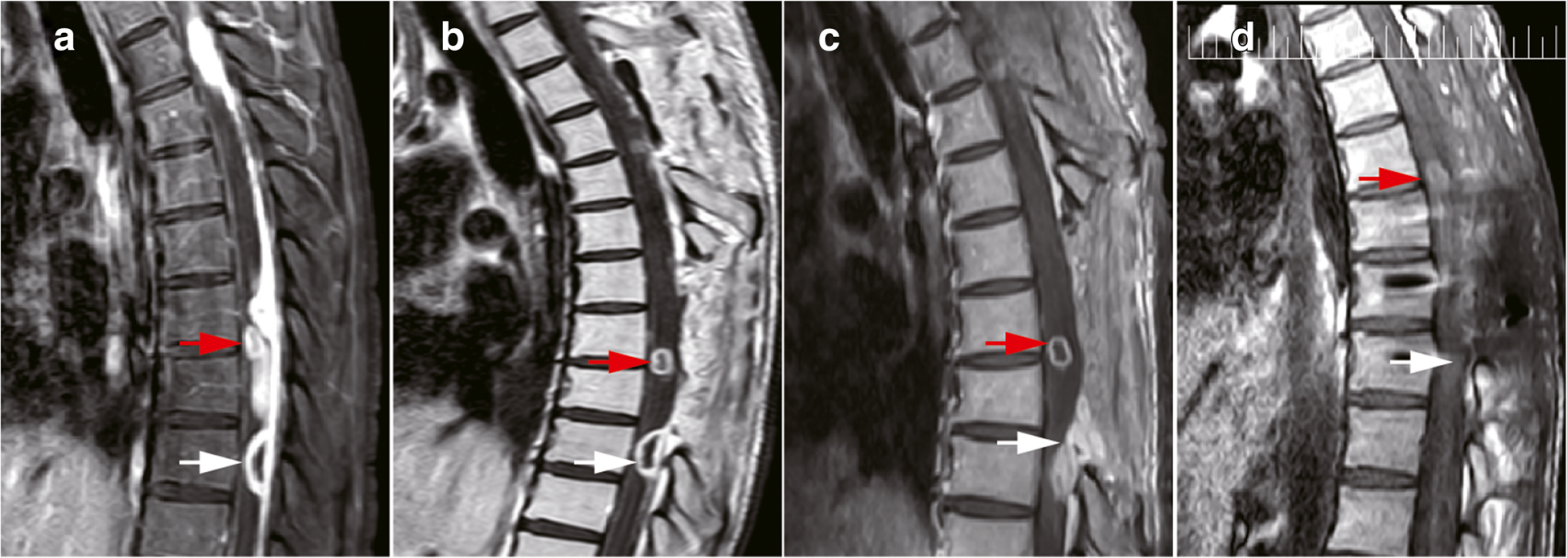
MRI of a patient with intradural extramedullary tuberculoma and intramedullary tuberculoma (two operations). a Preoperative MRI of the first operation of the patient manifesting intradural extramedullary tuberculoma from T3 to T9 (the enhancing lesion from T3 to T9) and intramedullary tuberculoma (red arrow). b Postoperative MRI of the first operation of the patient manifesting intradural extramedullary tuberculoma from T3 to T9 and intramedullary tuberculoma (red arrow). The intramedullary tuberculoma of T7 was left untouched while the intradural extramedullary lesion of T9 was missed (white arrow). c Preoperative MRI of the second operation of the patient. The intradural extramedullary tuberculoma of T9 was the target of operation (white arrow). d Postoperative MRI of the second operation of the patient. The intradural extramedullary tuberculoma of T9 was excised (white arrow)
